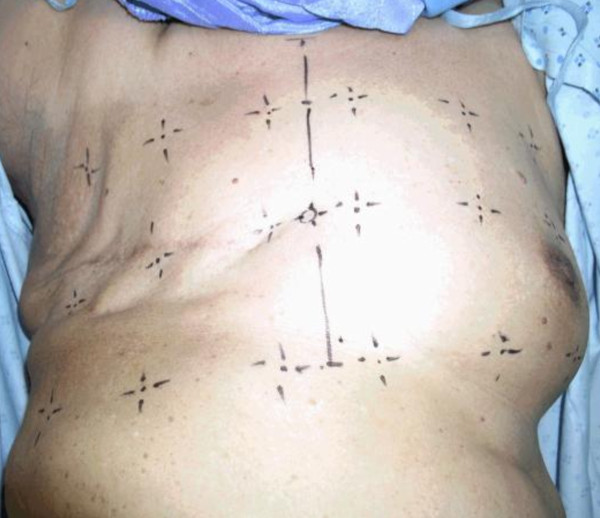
Points marked on the chest wall and contra-lateral non-irradiated breast prior to ultrasound.
